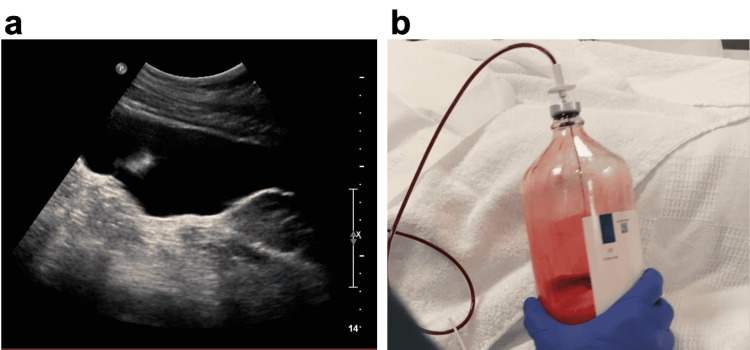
IR-guided paracentesis: an ultrasonographic image of ascites (a) and draining frank blood (b)
